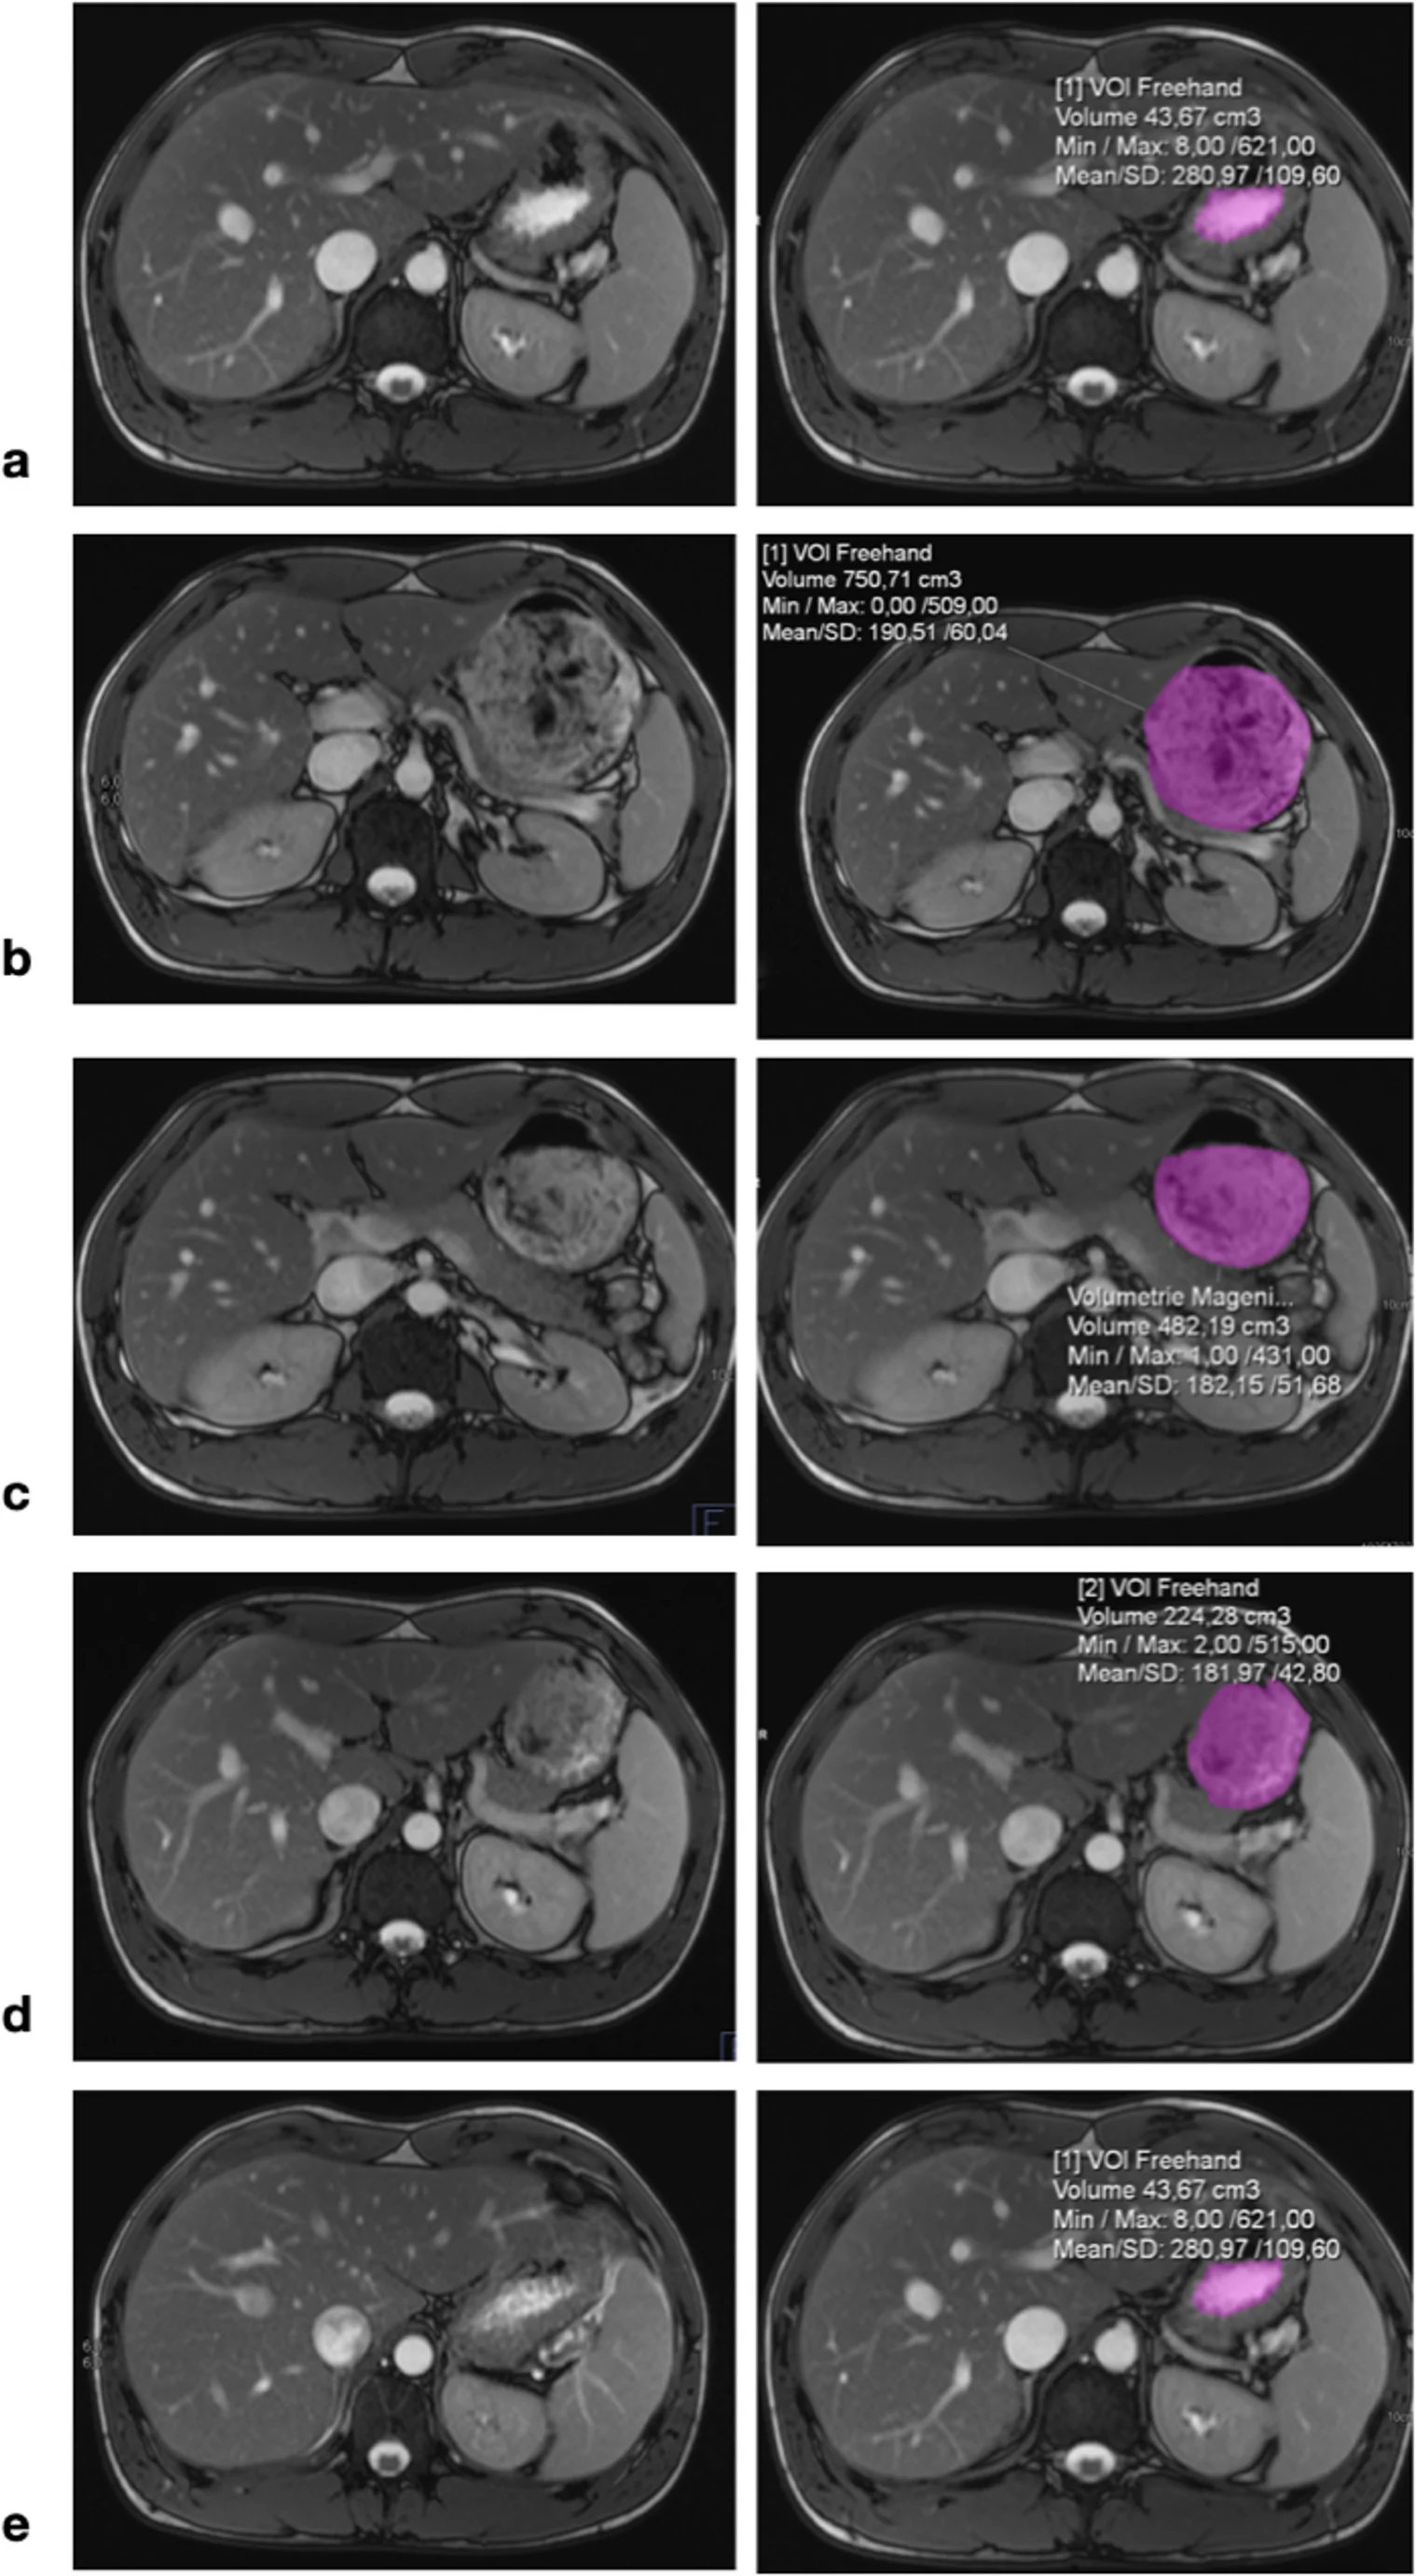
Gastric content assessment by MRI in the axial plane of the TRUFI-sequence. Shown is the gastric content after consumption of the high-fat meal. Even without contrast medium, gastric content shows sufficient contrast for volume determination. MRI-scan (a) after 12 h of fasting, (b) directly after meal consumption, (c) after 120 min, (d) after 240 min, (e) after 360 min
